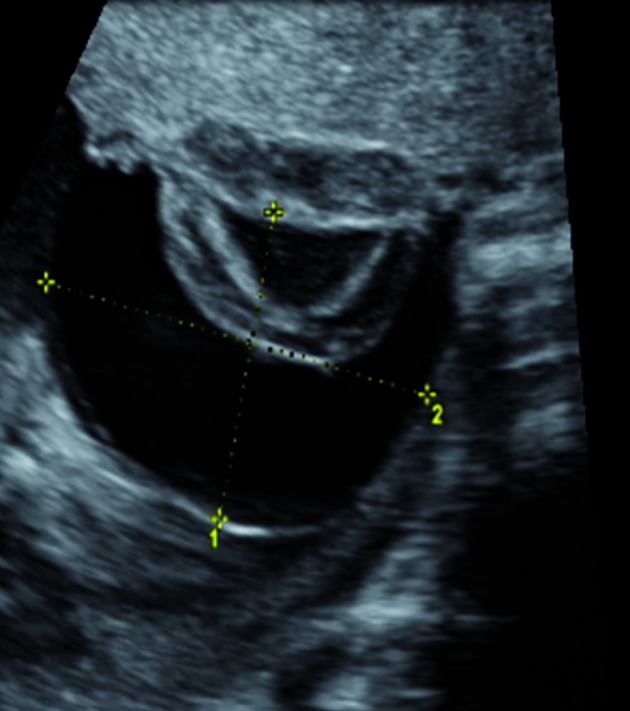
Ultrasound of one of the cysts, measuring 5 cm, located at the fetal placental side and containing echogenic material suggestive of hemorraghe.
